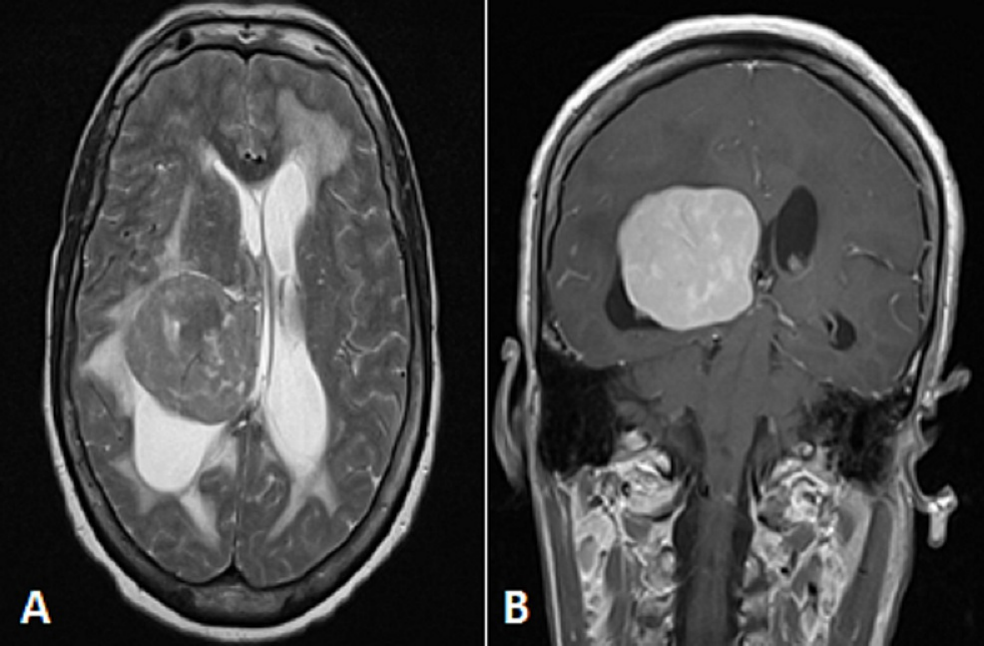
MRI brain with gadolinium. A: T2- weighted imaging (axial view) showing heterogenous isointense mass within the right lateral ventricle B: T1- weighted imaging (coronal view) showing contrast enhancement of tumour, with associated midline shift and obstructive hydrocephalus.
